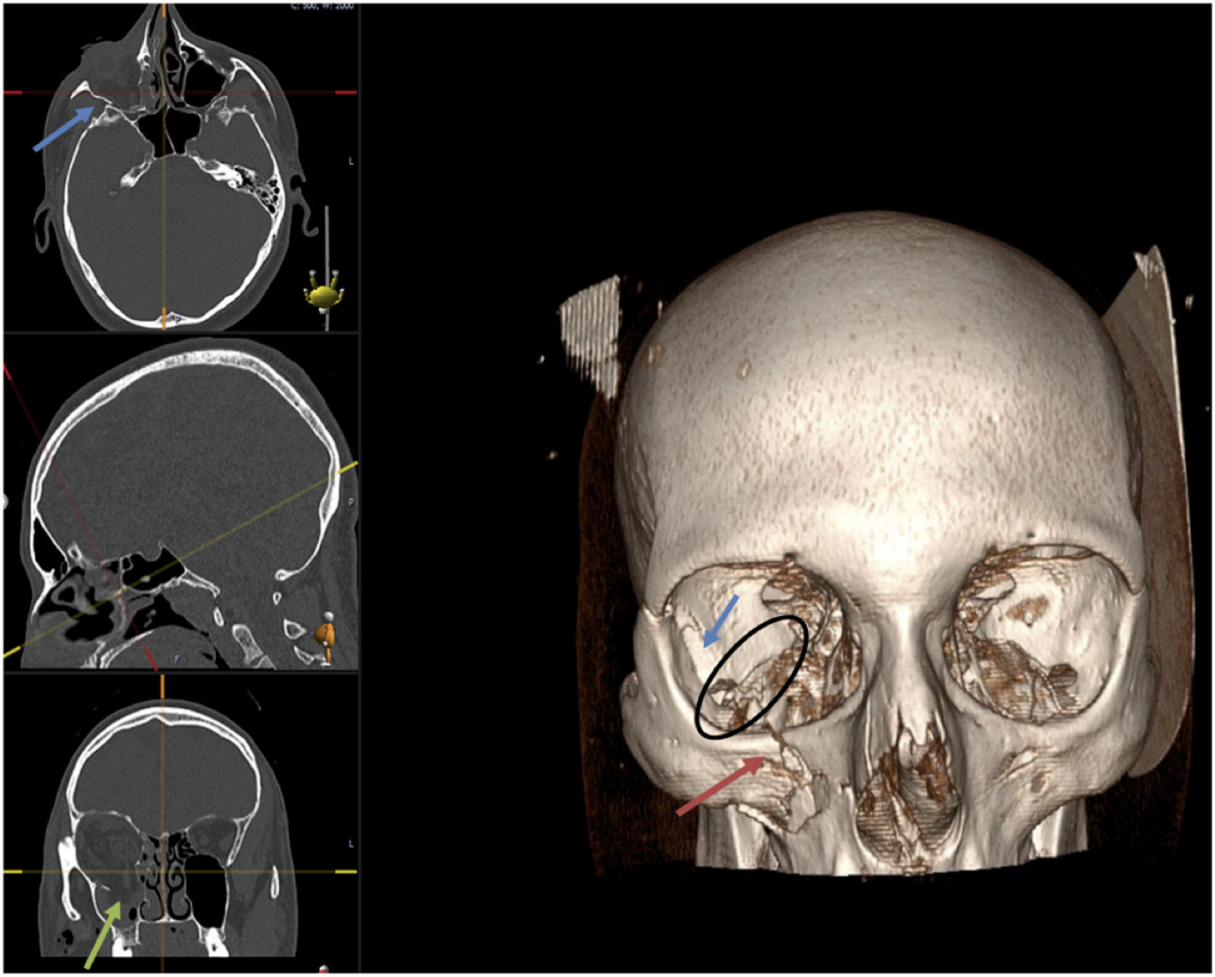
Facial bones CT demonstrating right orbital floor fracture (green arrow), lateral wall fracture (blue arrow) with the involvement of the infra-orbital rim (red arrow). The black circle shows the inferior orbital fissure (IOF) involved in the fracture on the right side compared to the left orbit.
